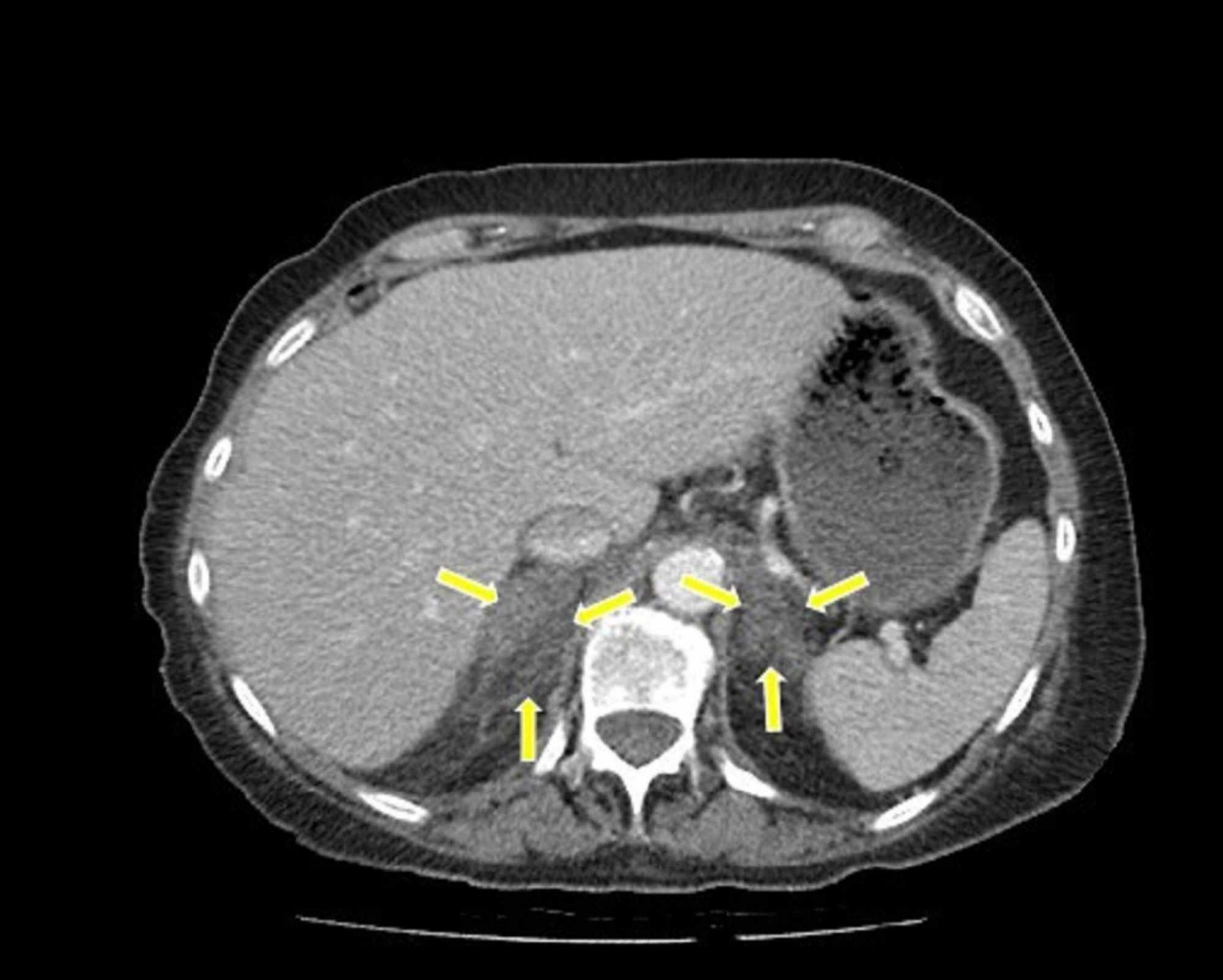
CT scan demonstrating bilateral adrenal hemorrhage (arrows)
